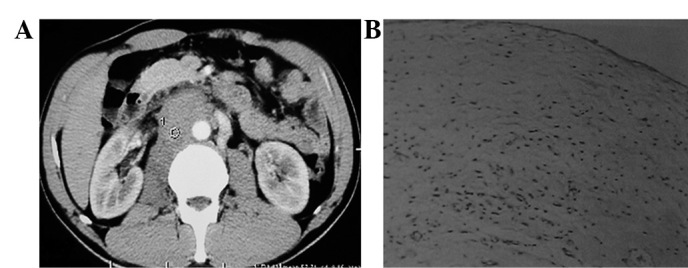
(A) CT scan of the abdomen showing the retroperitoneal mass on the plane of the right renal vein. The inferior vena cava was surrounded by the mass. (B) Mass biopsy with retroperitoneal laparoscopy showing fiber and fat tissues infiltrated by inflammatory cells.
